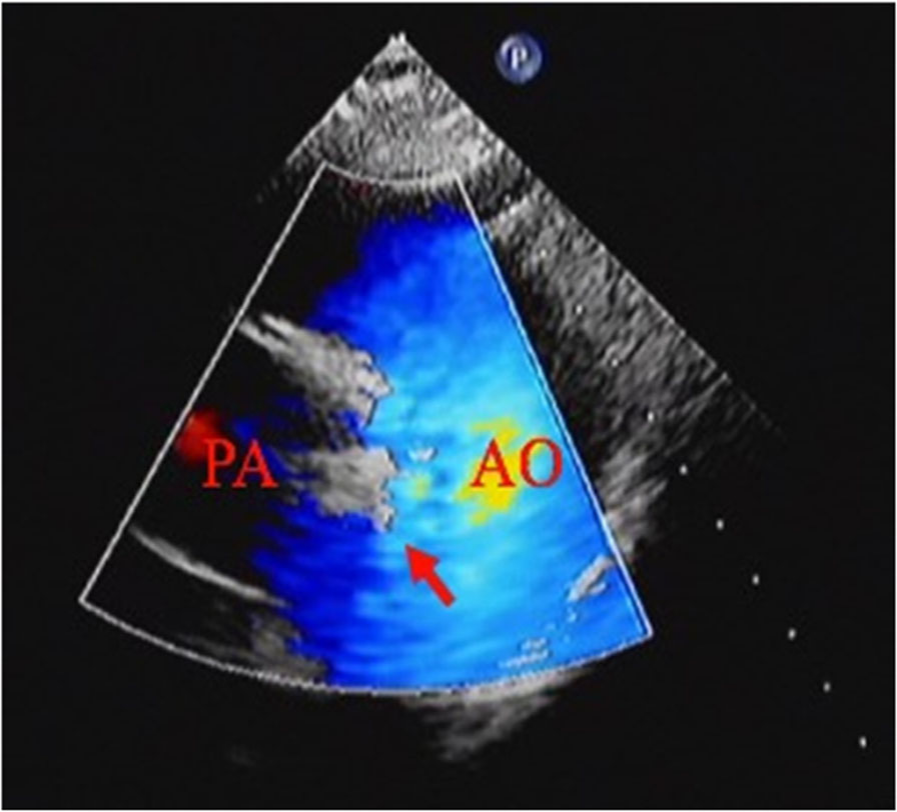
Transthoracic echocardiography results after occlusion. The red arrow indicates the occluder. There was no residual shunt after occlusion. PA, pulmonary artery; AO, aorta
